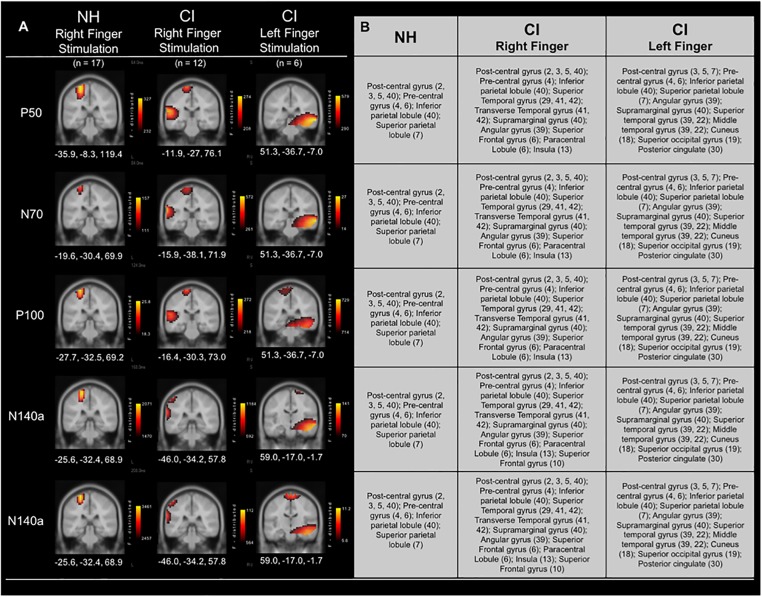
Current density reconstructions (CDR) for cortical somatosensory evoked potentials (CSEP) in normal hearing and cochlear-implanted children. (A) Cortical activations in response to vibrotactile stimulation of the right index finger in children with normal hearing (NH – left panel; *n* = 35) and cochlear implants (CI – middle panel; *n* = 12). Additionally, cortical activations in response to stimulation of the left finger in a subset of CI children who received their initial CIs in the right ear are shown in the right-most panel (*n* = 6). Activations are organized in rows corresponding to each CSEP waveform component (P50, N70, P100, N140a, N140b). CDRs are presented on coronal slices for each of these components. Three-dimensional Montreal Neurological Institute coordinates for each activation are listed below each MRI slice. The *F*-distribution scale (bottom) presents the color gradient associated with the maximum (yellow) through the minimum (black) likelihood for activation as calculated by sLORETA. (B) A table listing all areas of significant activation for each CSEP waveform component. Brodmann areas are indicated in parentheses.
